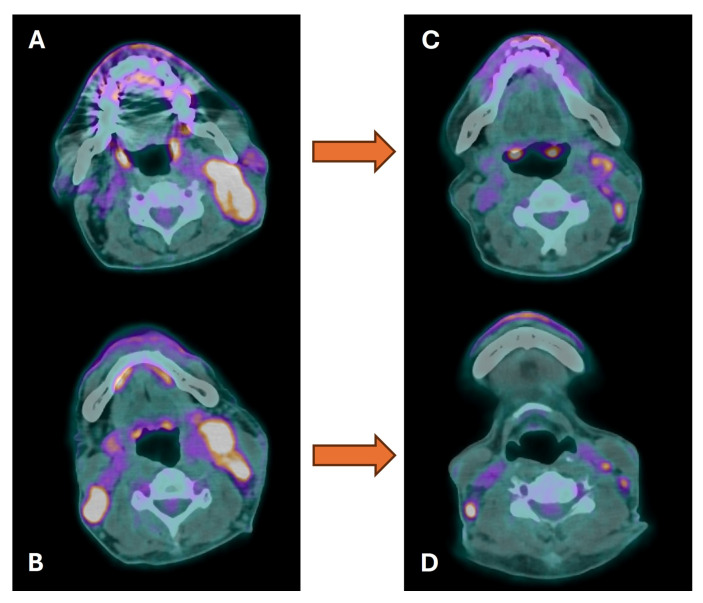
Baseline (A,B) and post-chemotherapy (C,D) ^18^F-FDG PET/CT in a patient with bilateral cervical lymph node metastases from nasopharyngeal carcinoma. Marked reduction in entity and extent of ^18^F-FDG, as well as in size, was observed in all the involved lymph nodes, with a persistent inhomogeneous uptake and no evidence of new active lesions (partial response).
